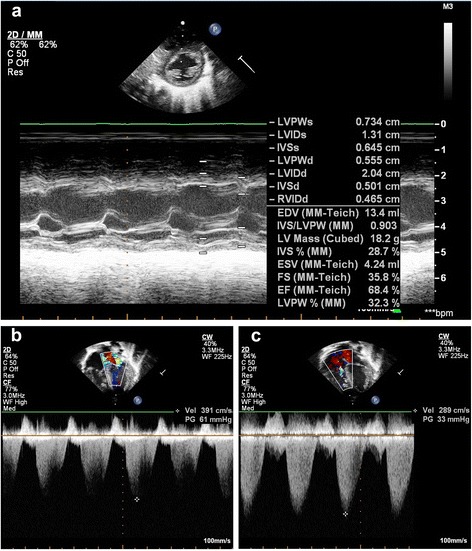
Echocardiography of a Sengers patient. a. M-Mode of LV in short axis showing dilated LV for age of (Case-3) at 6 days (LVEDD 2.04). b. moderate mitral valve regurgitation with left atrial contraction in systole. c. moderate tricuspid regurgitation with right atrial contraction envelope in systole.
